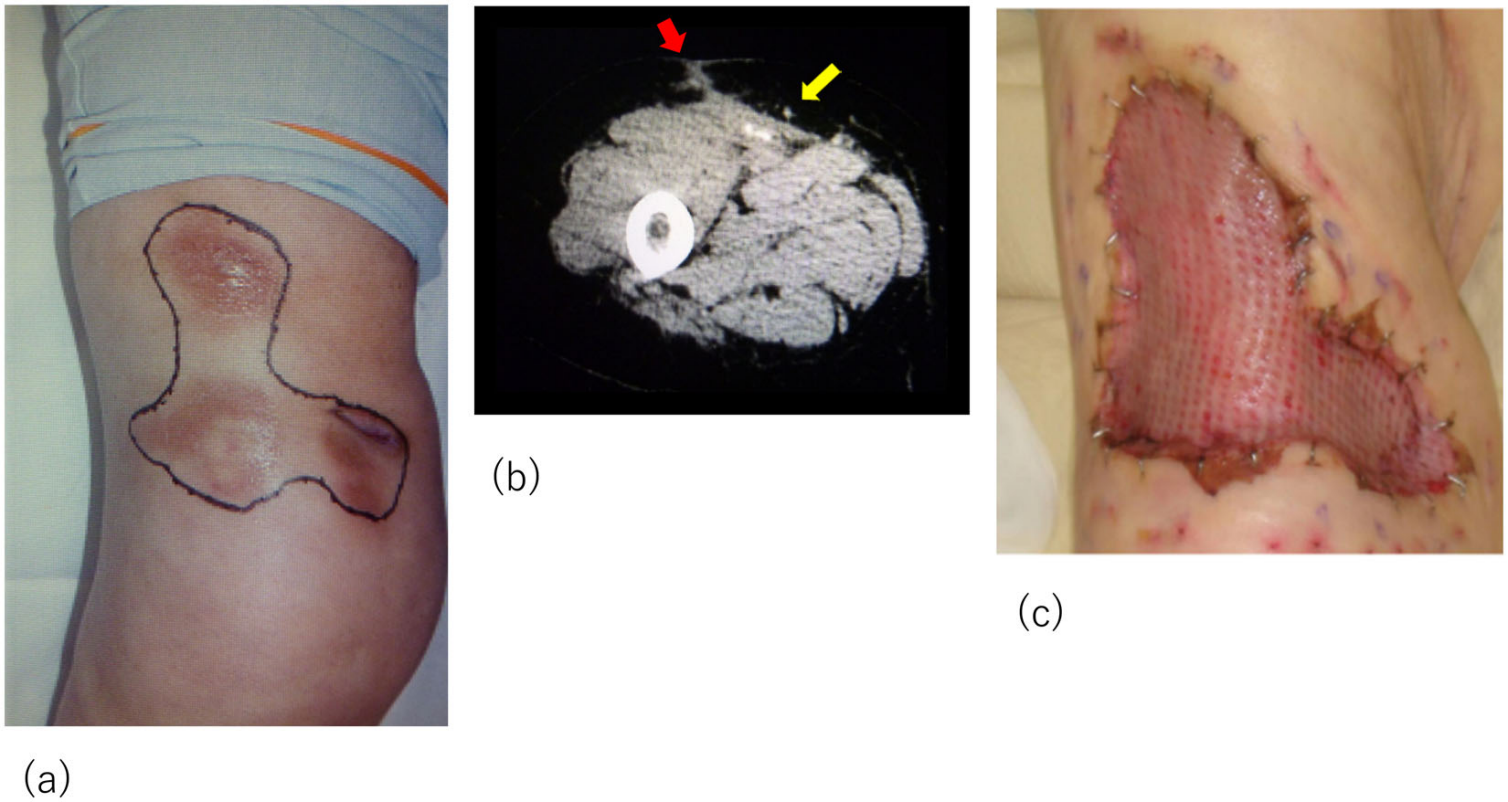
Recurrent lesion in the middle of the right thigh. (a) The lesion in the middle of the right thigh; obvious nodules with redness are marked. (b) Computed tomography images of the lesion: yellow arrow indicates the calcification in the muscle and red arrow indicates inflammation spreading towards subcutaneous tissue. (c) Meshed split-thickness skin graft taken successfully.
